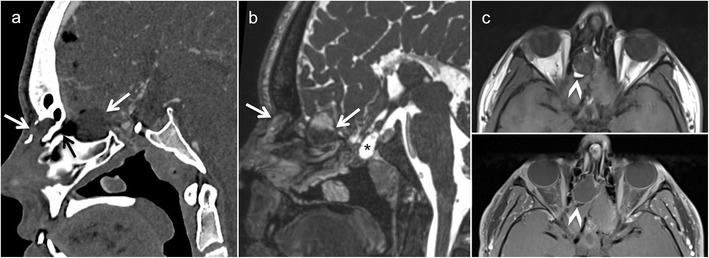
Nasal dermoid sinus cyst (NDSC) visible since birth in a 15-year-old boy. a Sagittal contrast-enhanced CT scan (soft tissue windows) shows the extra- and intracranial dermoid cysts (*white arrows*), which connect with each other via the abnormal foramen caecum (*black arrow*). b High-resolution sagittal T2 shows that the cystic lesions have different signal intensities (*arrows*), suggesting different proteinaceous/lipomatous contents. Note also corpus callosum agenesis and posterior ethmoidal meningocele (*asterisk*). c The lipomatous content (*arrowheads*) of the NDSC appears hyperintense on T1 (*upper image part*) and hypointense on fat-saturated contrast-enhanced T1 (*lower image part*), respectively
